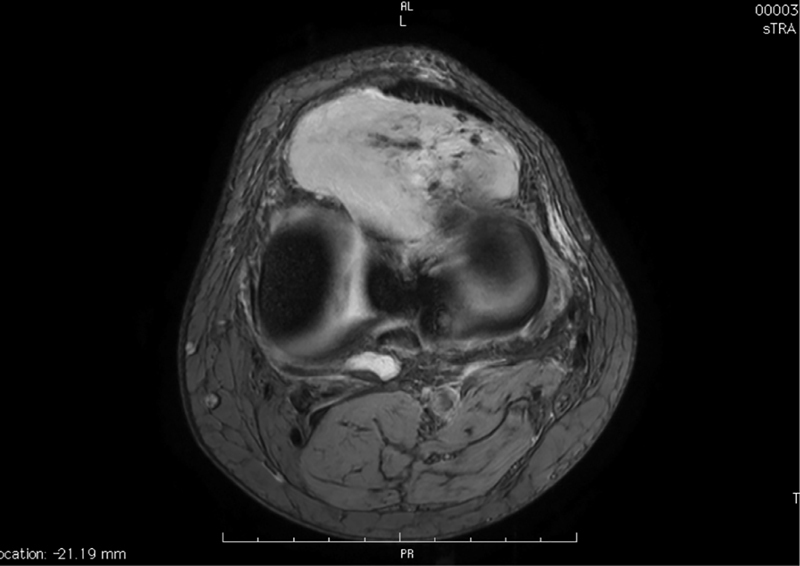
Coronal T2-weighted MRI showing a well-circumscribed schwannoma. MRI, magnetic resonance imaging.
